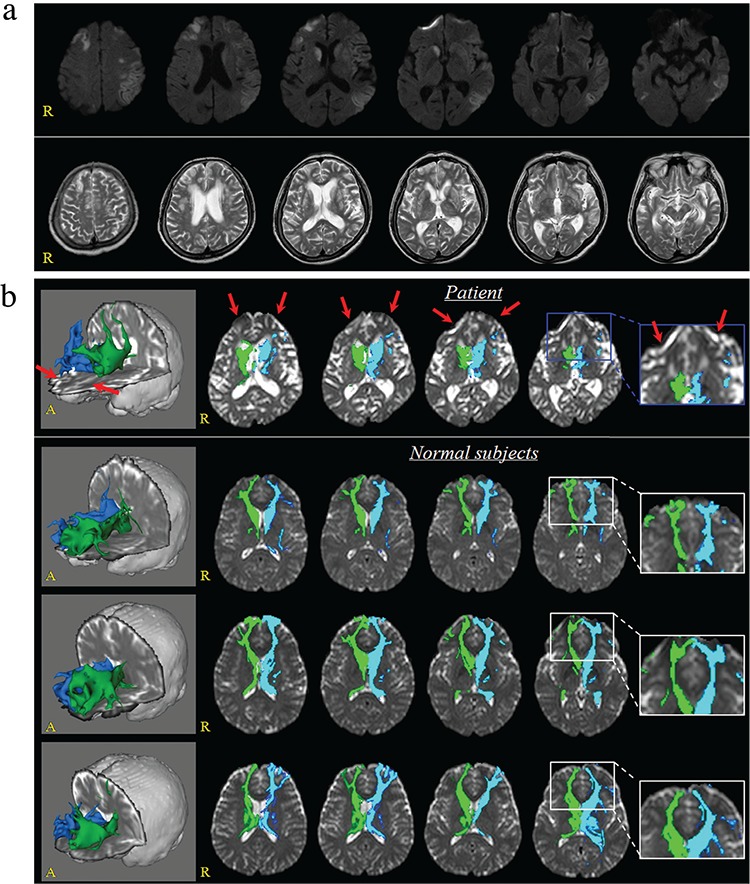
Diffusion (2 days after onset, upper low) and T2-weighted (4 weeks after onset, lower low) brain MR images showing multiple infarcts in the bilateral caudate nucleus, the bilateral frontotemporal lobes, and the left parietal lobe (a). The neural connectivity of the caudate nucleus to the prefrontal cortex (red arrows) is absent in both hemispheres compared to that in three normal subjects (three females, mean age: 62.3±3.5 years) (b).
